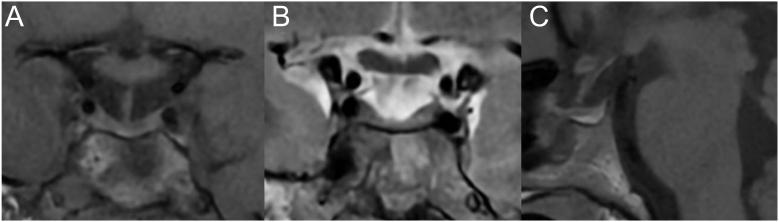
Postoperative pituitary MRI performed without contrast at 27, 5 weeks of pregnancy showing complete resection. (A) Coronal T1-weighted image. (B) Coronal T2-weighted image. (C) Sagittal T1-weighted image.
